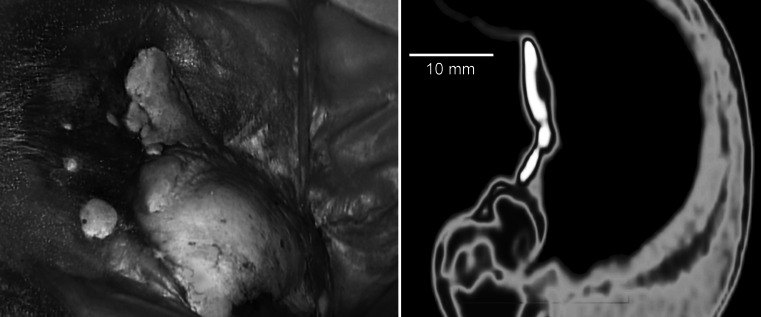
Intracranial view of the calcified spots of the dura mater adjacent to posterior wall of the orbit afflicted with a tumor. CT scan presents calcification of the dura mater
